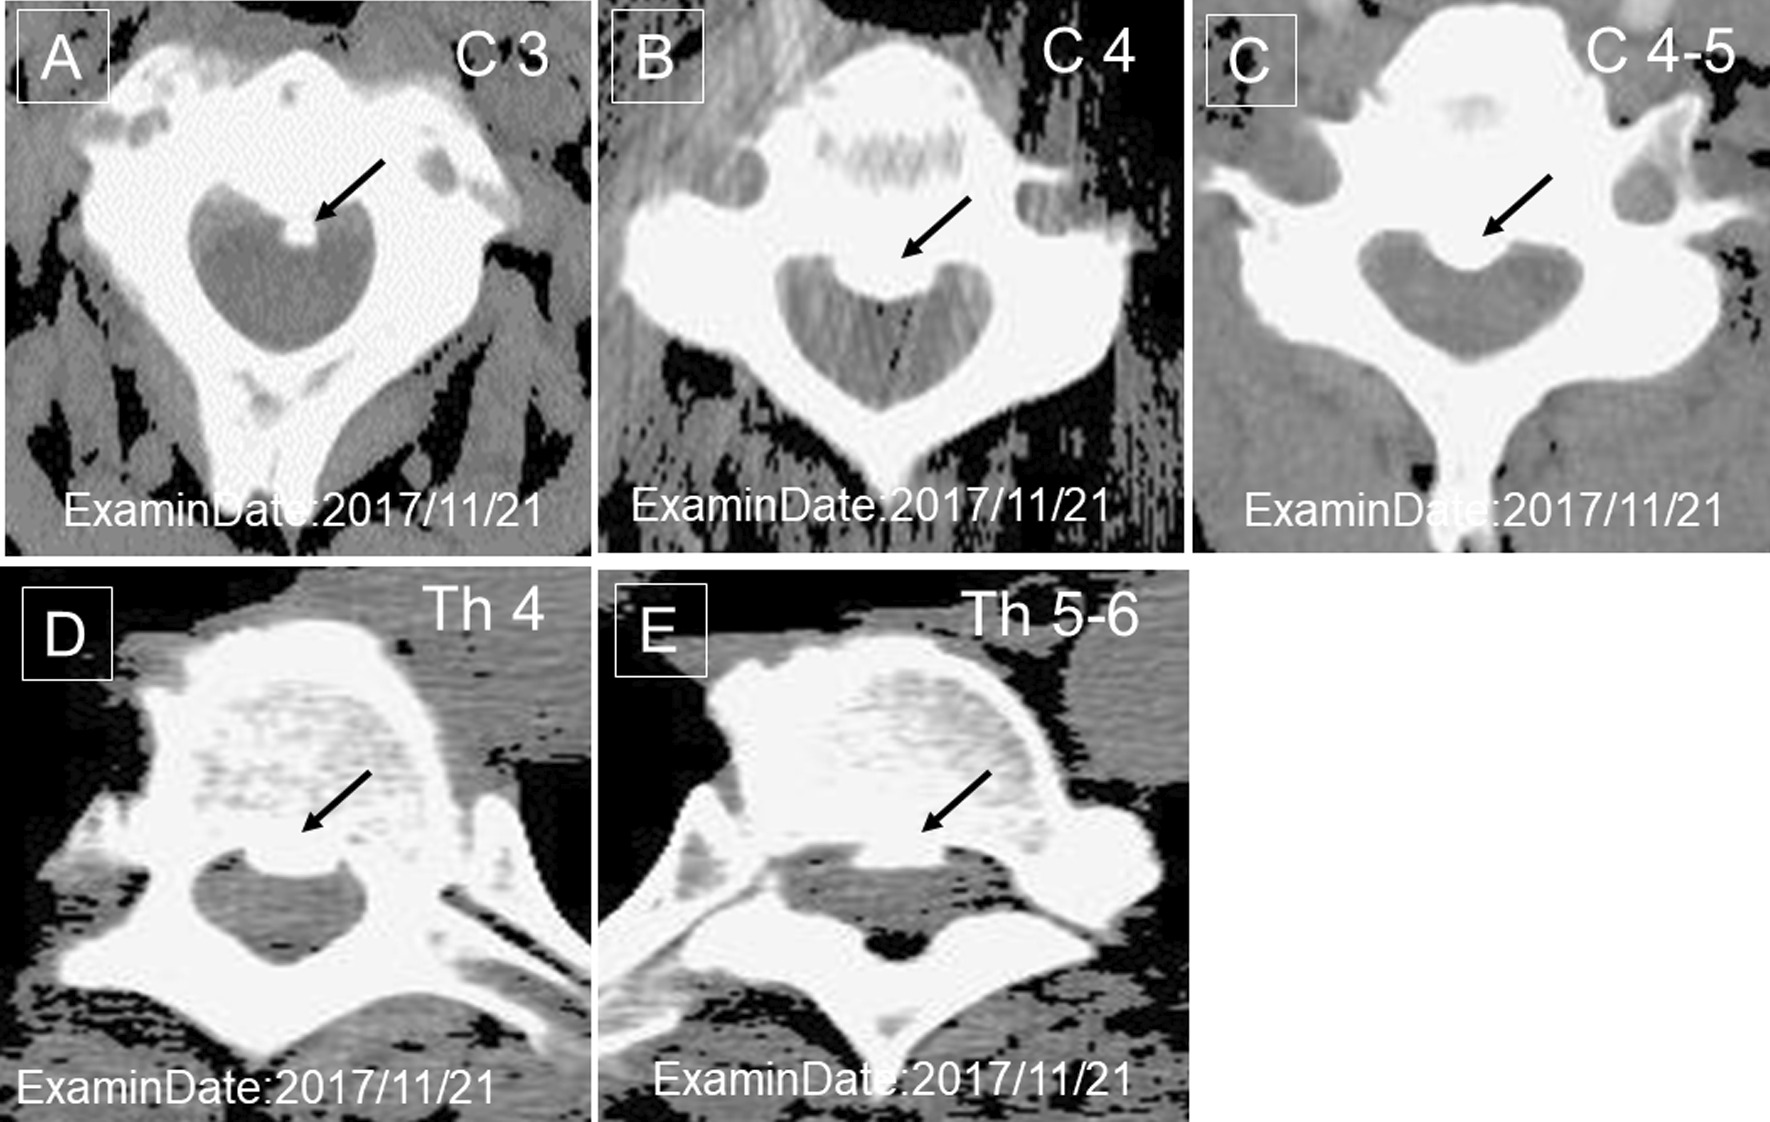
Computed tomography images of the ossification of the posterior longitudinal ligament of the cervical thoracic spine on 21 November 2017. a The arrows indicate the ossification of the posterior longitudinal ligament of the third cervical vertebra, b the fourth cervical vertebra, c the third to the fourth cervical vertebrae, d the fourth thoracic vertebra, and e the fifth to the sixth thoracic vertebra
